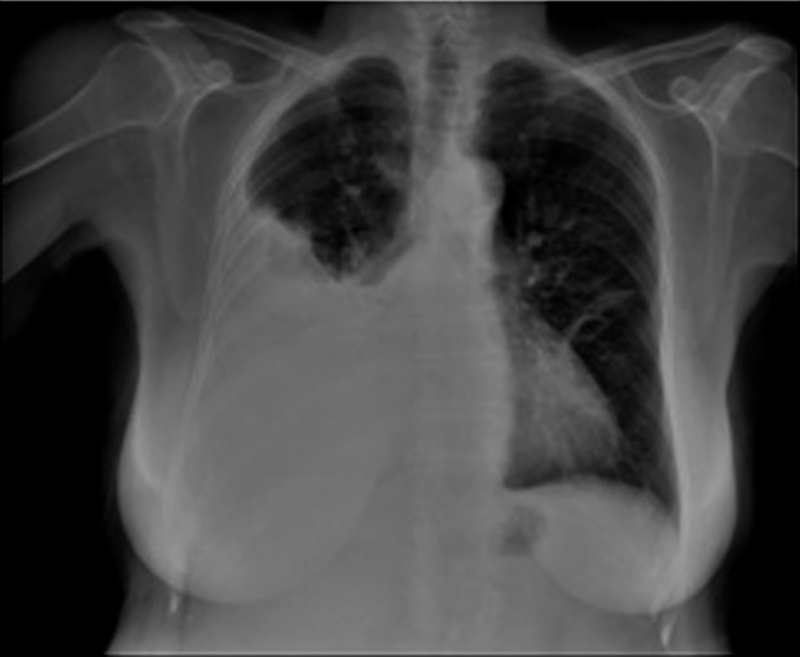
Posteroanterior chest X-ray showed increase in density in the lower zone of the right hemithorax .
